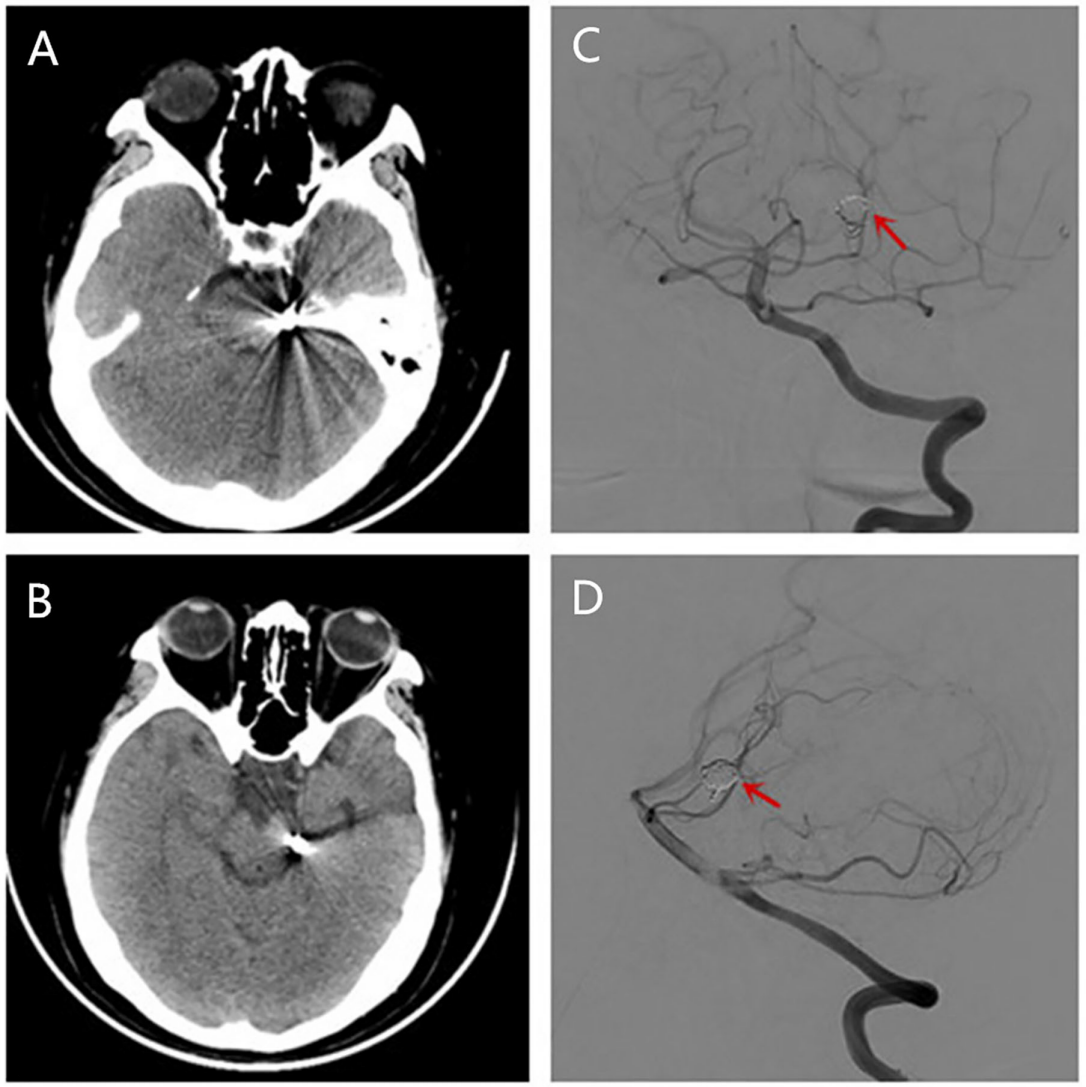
Brain CT (A,B) conducted showing no localized hemorrhage or ischemic lesions after she had a migraine-like headache. Reexamination of DSA showing the coil was stable and there was no *in situ* thrombus (C,D).
